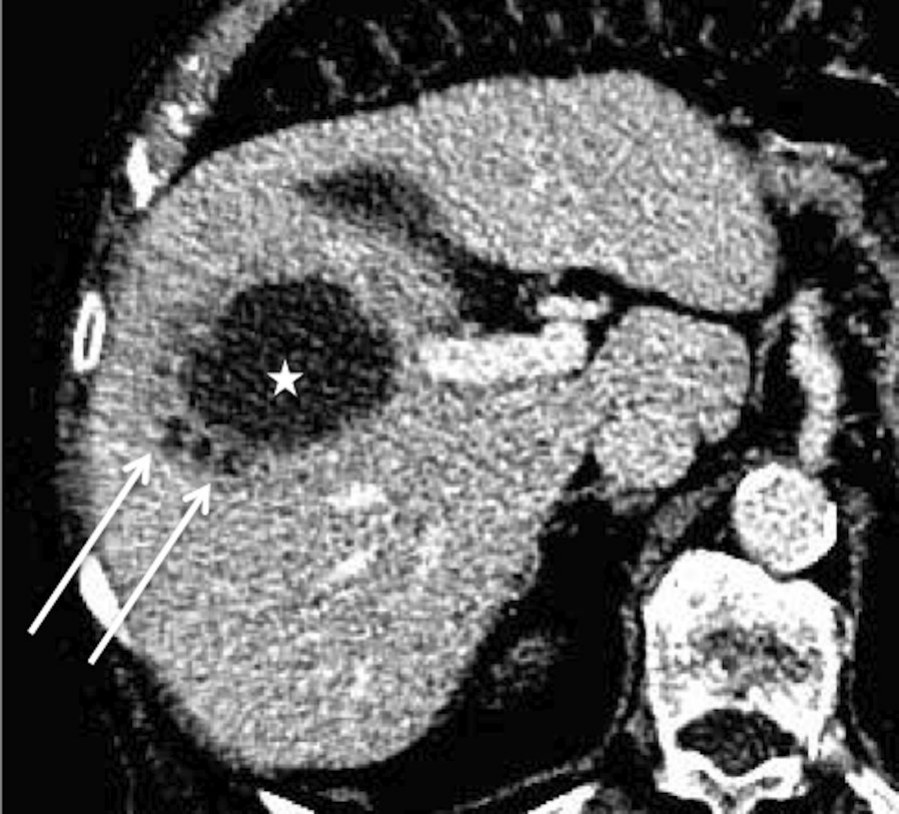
Escherichia Coli* abscess in an 89-year-old male. Axial computed tomography on portal venous phase shows three small hypoattenuating areas (arrows) forming the “cluster sign” and a large central hypoattenuating cavity (star)
